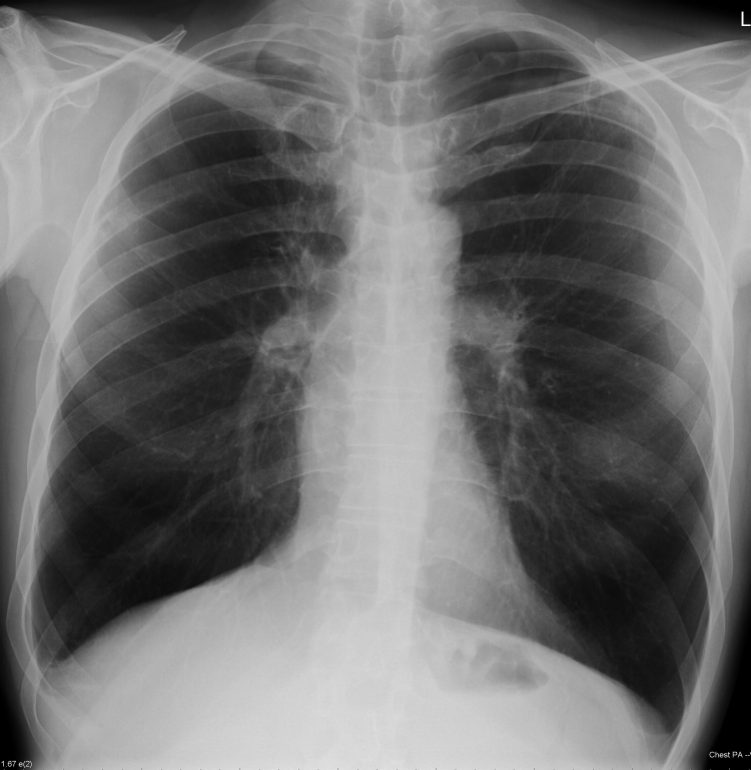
Patient 1 Chest radiograph 3 years later, showing generalised emphysema but no recurrence of chronic pulmonary aspergillosis
